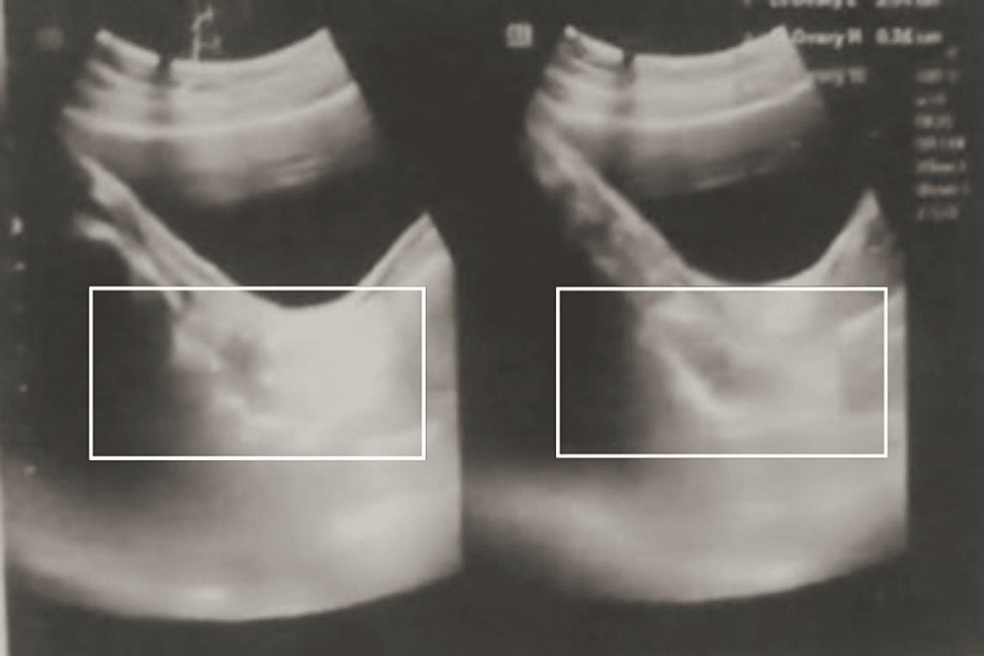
Ultrasonography showing the hypoplastic uterus
